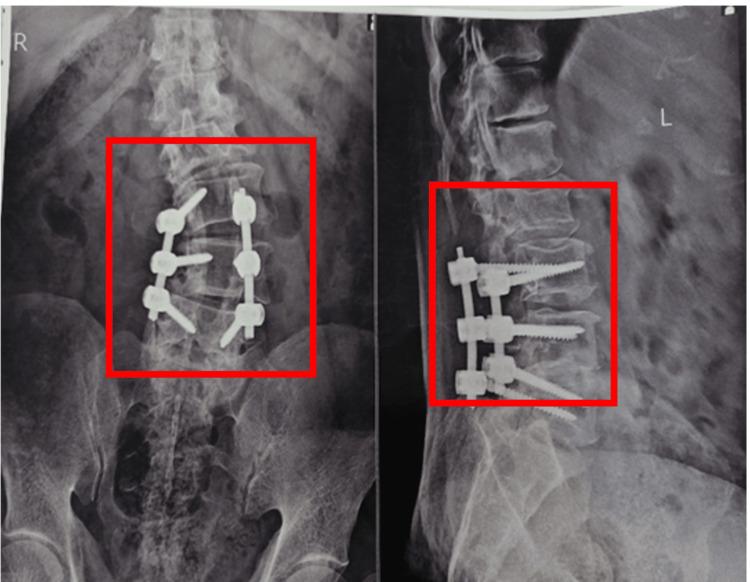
The post-operative X-ray of the lumbar spine (red squares)
